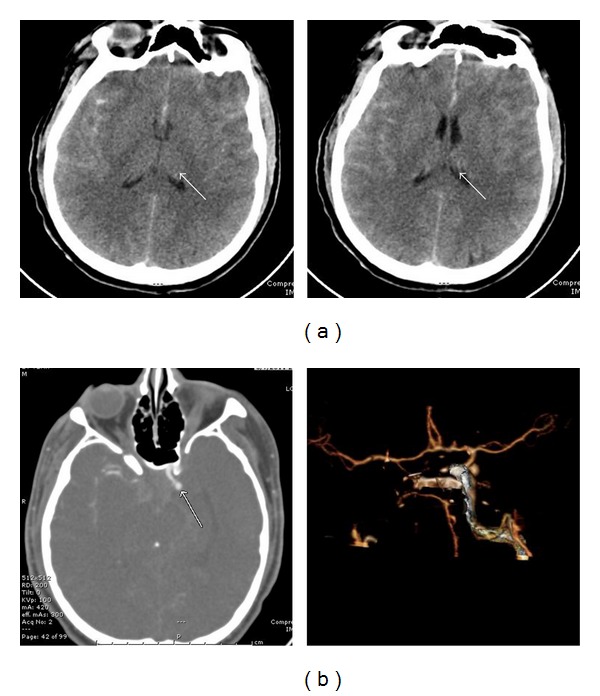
Imaging studies showing spontaneous subarachnoid hemorrhage. (a) CT head without contrast showed subarachnoid hemorrhage with intraventricular involvement. (b) CT angiogram of brain demonstrated a 4-5 mm saccular left posterior communicating artery aneurysm.
